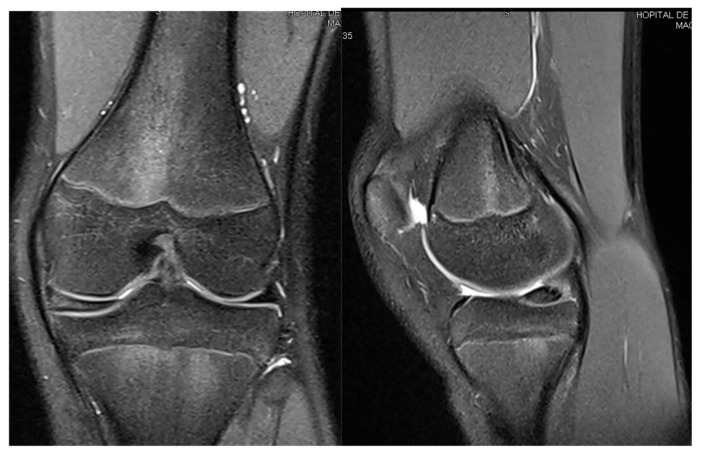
Patient G.L. (14 years old). MRI of the left knee in a patient with bilateral symptoms of medial discoid meniscus (left—coronal T2 view; right—sagittal T2 view). The coronal view revealed a widened medial meniscus with an abnormal bow-tie appearance spanning more than two contiguous slices, indicative of a discoid morphology. The sagittal image showed increased signal intensity within the posterior horn, suggesting possible early tearing or degeneration. These findings supported bilateral involvement, although only the right knee underwent surgical treatment during the study period.
